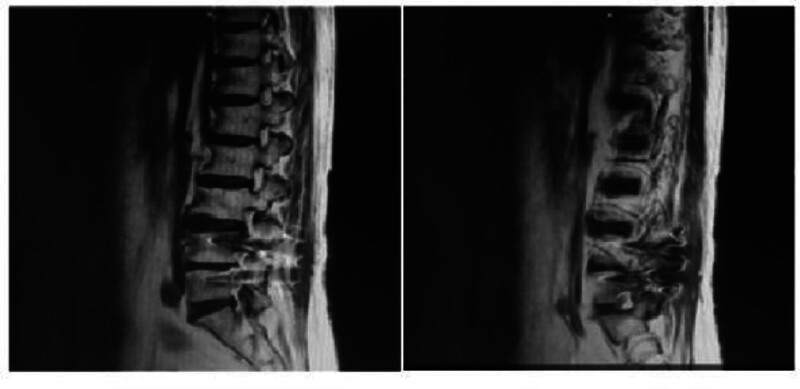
Preoperative magnetic resonance imaging revealed recurrent disc herniation at L3/4 as the primary pathological segment, with circumferential disc bulging noted from L1/2 to L5/S1.
